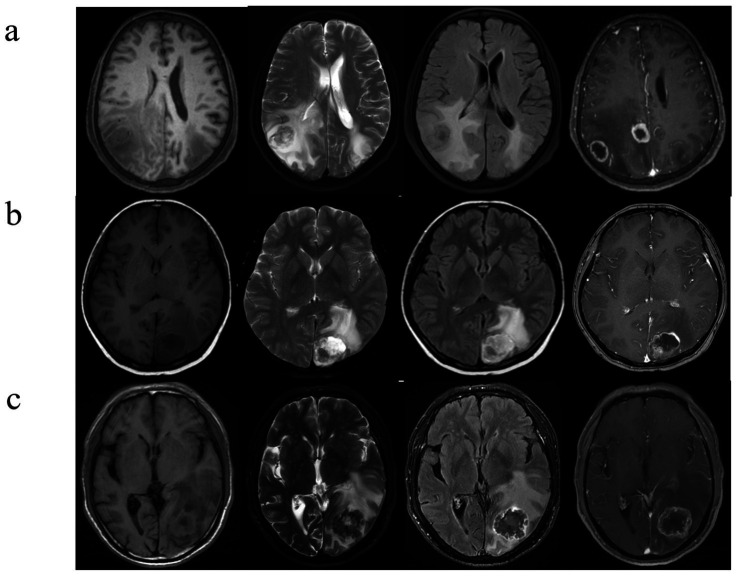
Brain metastases with atypical MRI findings. (A) A 73-year-old man with NSCLC. Multiple hyperintense nodules are observed in the bilateral occipital lobes and lateral ventricular trigones on T2WI and FLAIR sequences, accompanied by significant perilesional brain edema, and there is evident ring enhancement on CE- T1WI. (B) A 53-year-old woman with a history of breast cancer surgery 3 years ago presents with cystic-solid, lobulated nodules located beneath the cortical layer of the left occipital lobe. The nodules exhibit mixed high signal intensity on both T2WI and FLAIR sequences, along with surrounding peritumoral edema. CE- T1WI reveals significant and heterogeneous enhancement. (C) A 42-year-old man, diagnosed with signet ring cell carcinoma on gastroscopy, presents with severe headaches. T2WI shows a low signal intensity nodule in the left occipital lobe, with unclear borders. The lesion demonstrates central hypointensity and peripheral hyperintensity on FLAIR sequences, accompanied by significant peritumoral edema. Additionally, there is heterogeneous ring enhancement surrounding the lesion.
